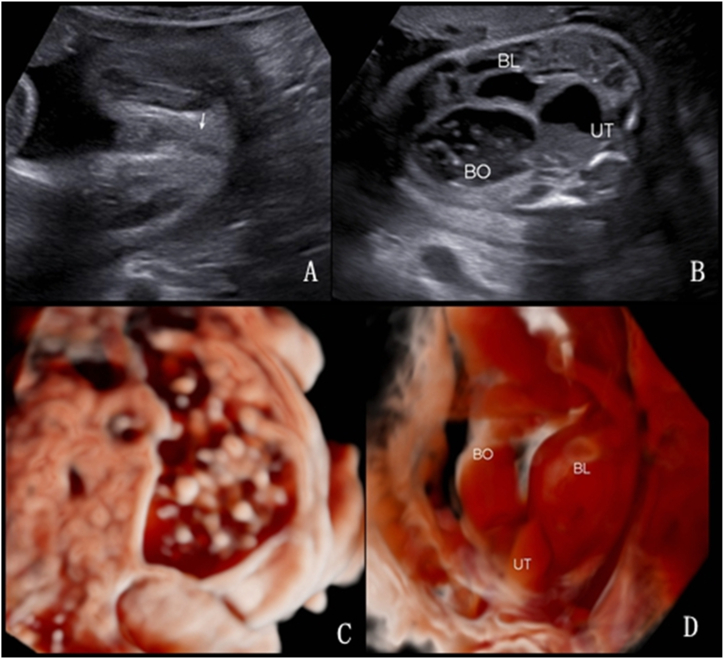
Urorectal septum sequence at 31 weeks and 6 days of gestation: A. 2D ultrasound shows absence of the “target sign” in the anal area; B. 2D ultrasound shows strong echo spots within the bowel, uterine distension with fluid accumulation, and local deposition of small echogenic spots; C. 3D imaging in grayscale mode demonstrates strong echogenic spots suspended within the dilated bowel; D. 3D imaging in inversion mode shows the spatial relationship between the bladder, uterus, and dilated colon, with the uterus appearing as a unicompartimental shape.
